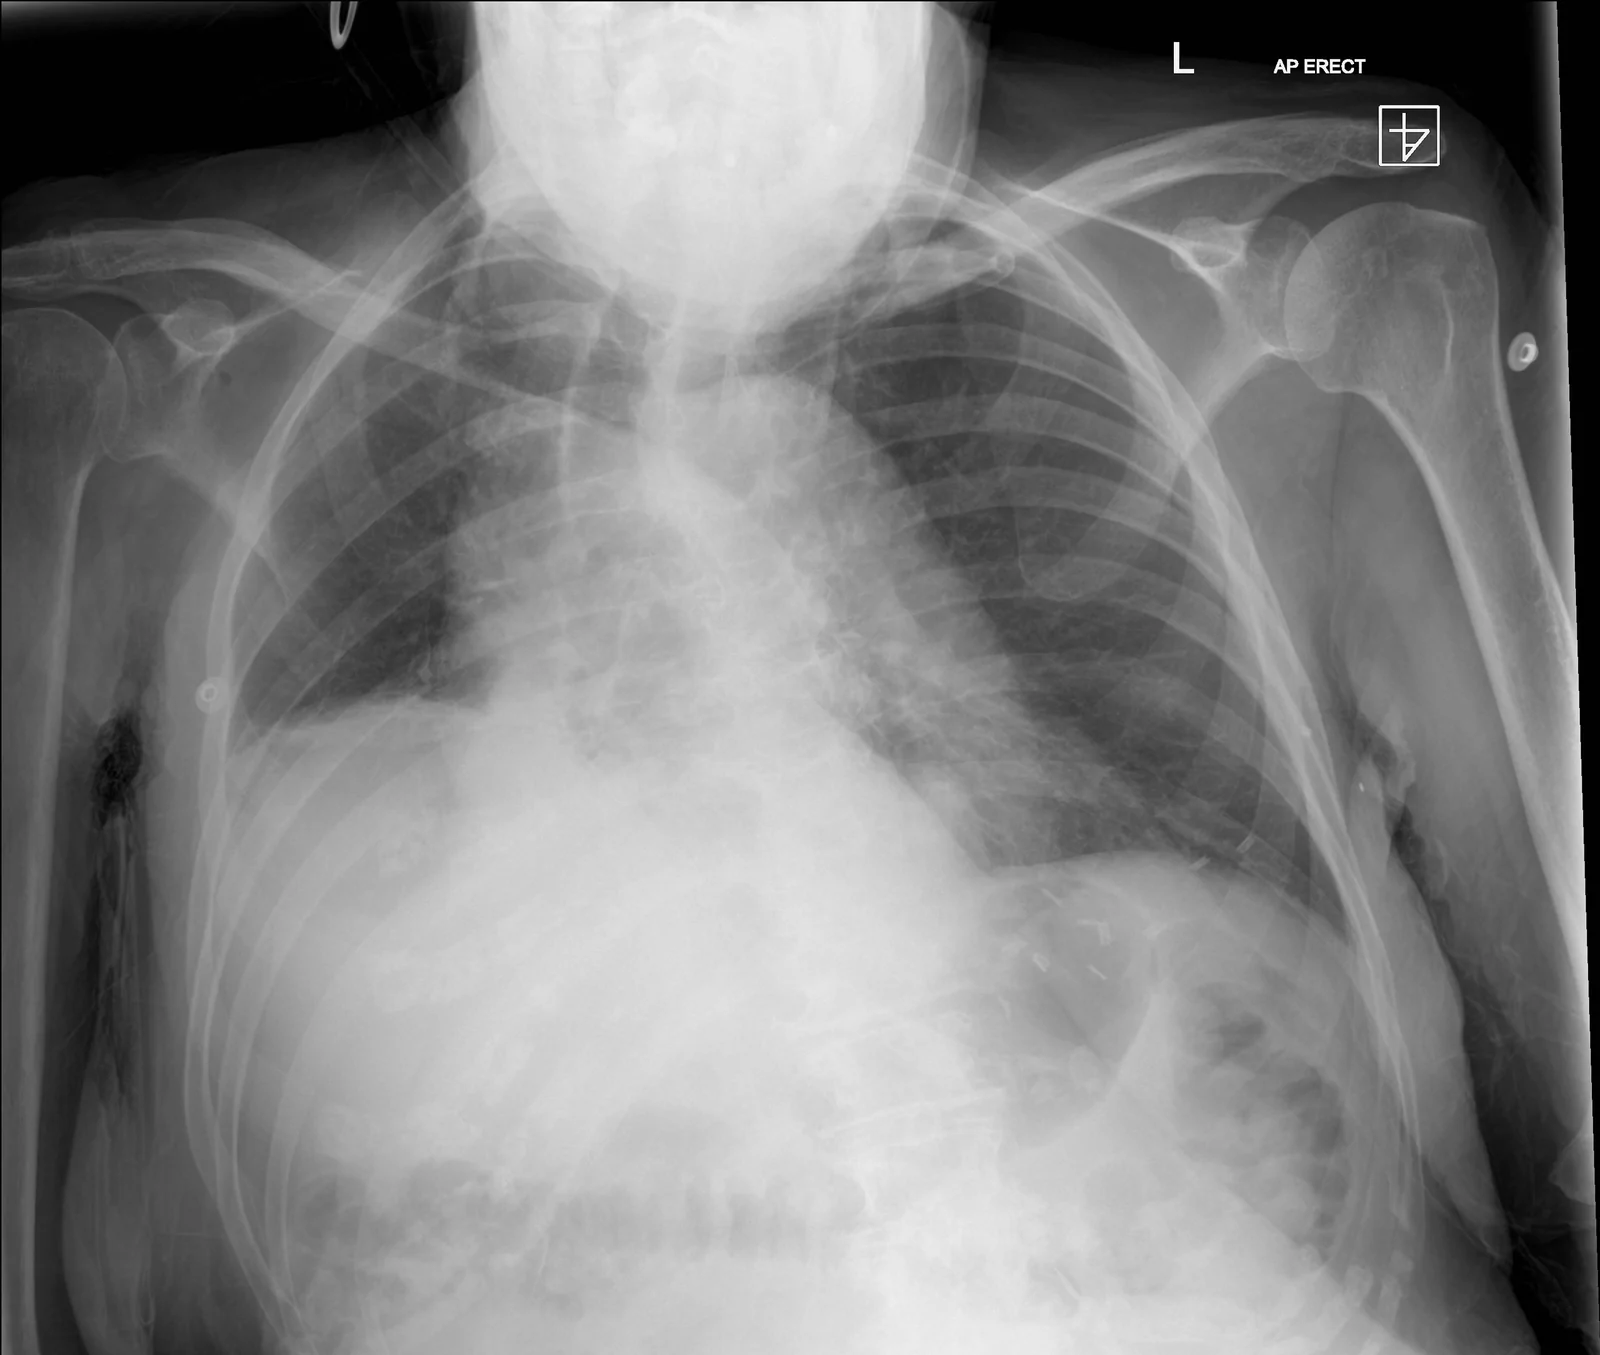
Chest X-ray showing a raised right hemi-diaphragm
